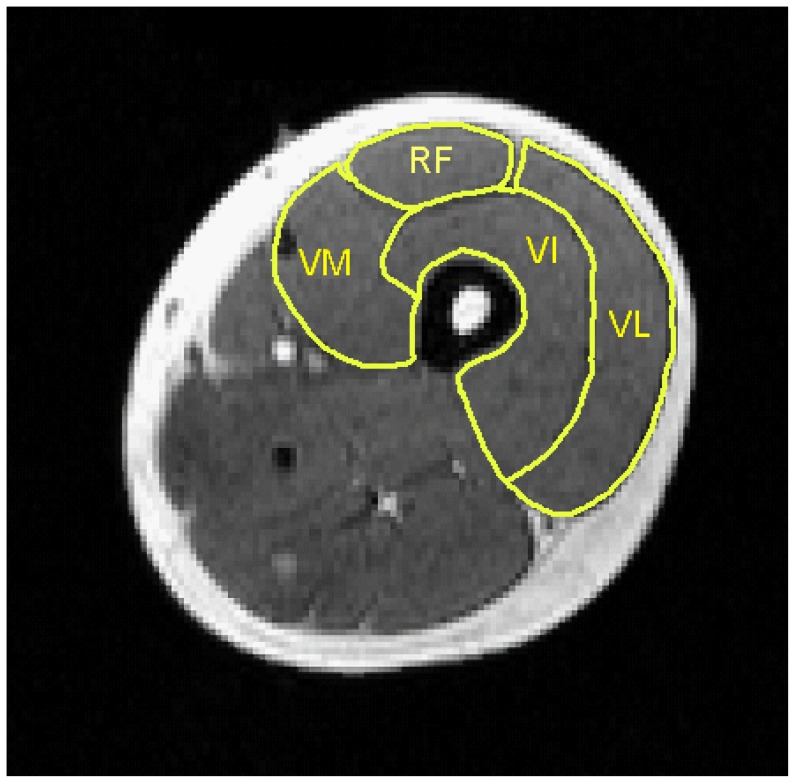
Regions of interest (ROI) drawn on MRI image example over the muscles constituting the quadriceps muscle. RF – m. rectus femoris, VL – m. vastus lateralis, VM – m. vastus medialis, VI – m. vastus intermedius.
